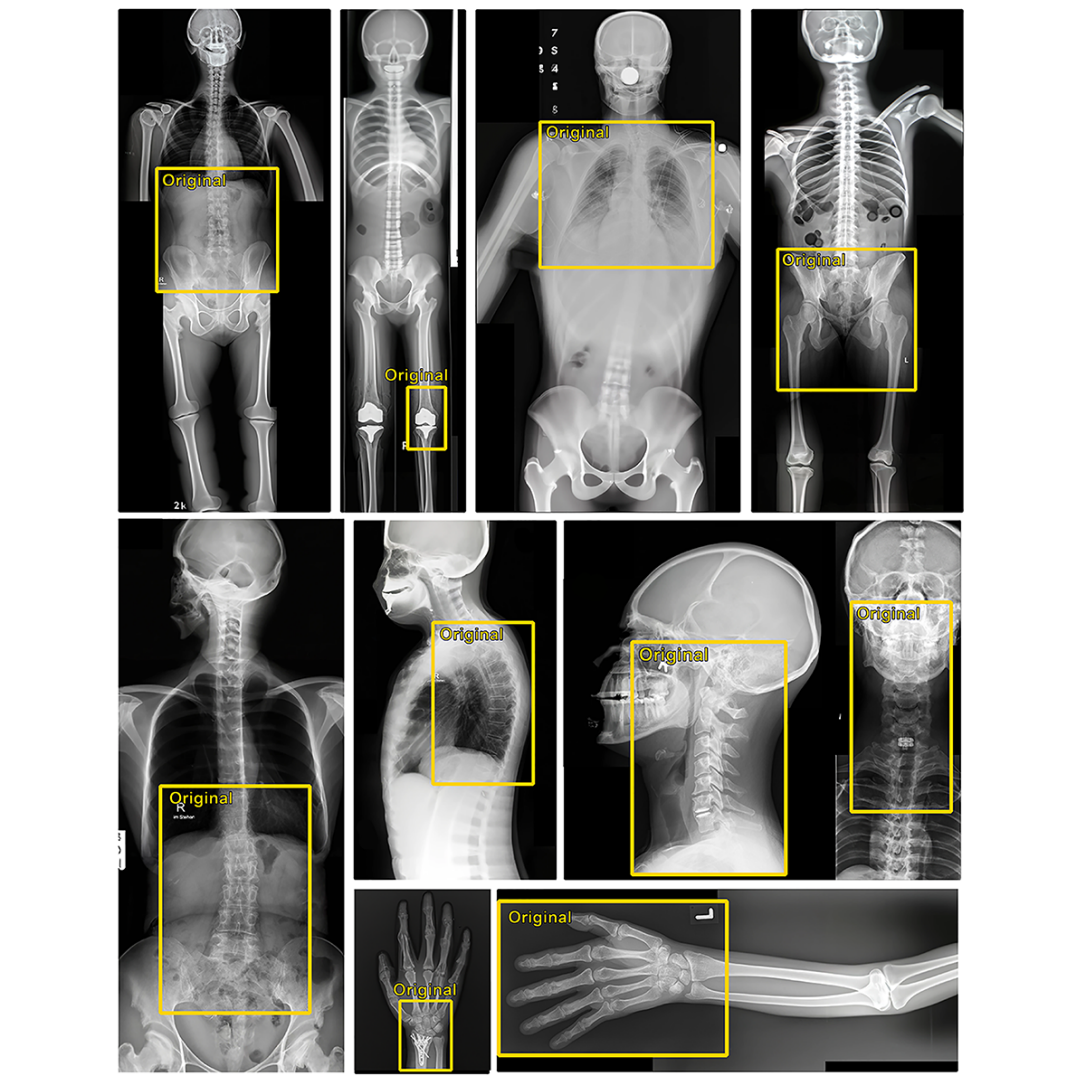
Extending x-ray images of different anatomical regions beyond their borders by using DALL-E 2. The original x-rays are shown in yellow boxes, and the areas outside of the yellow boxes were generated by DALL-E 2.
